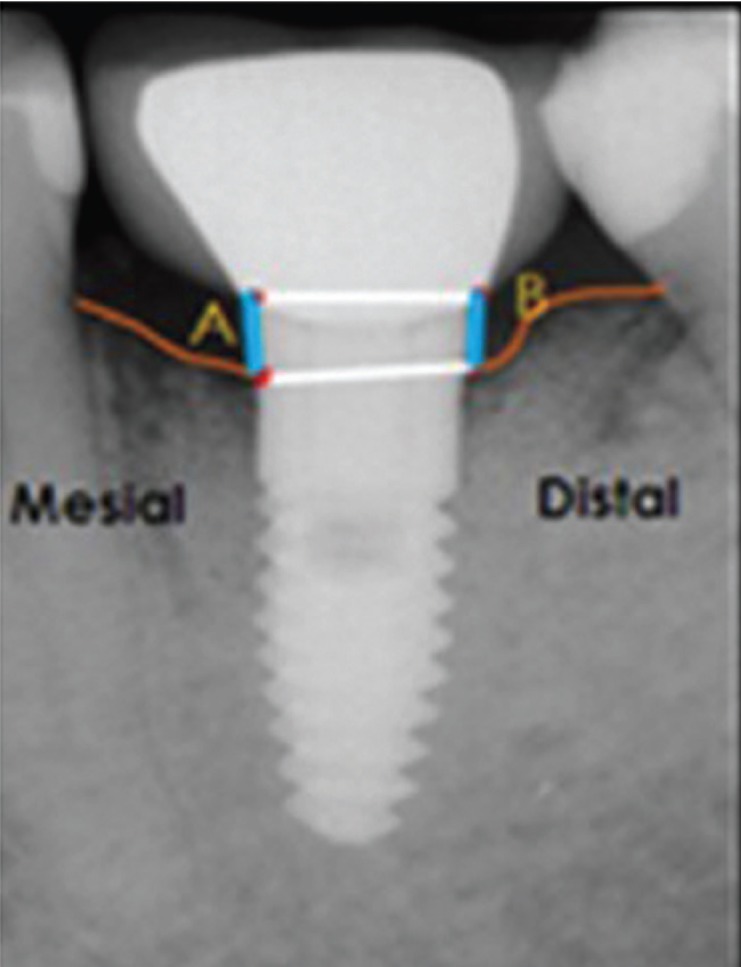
Periapical radiograph and the method employed to calculate the marginal bone loss.
